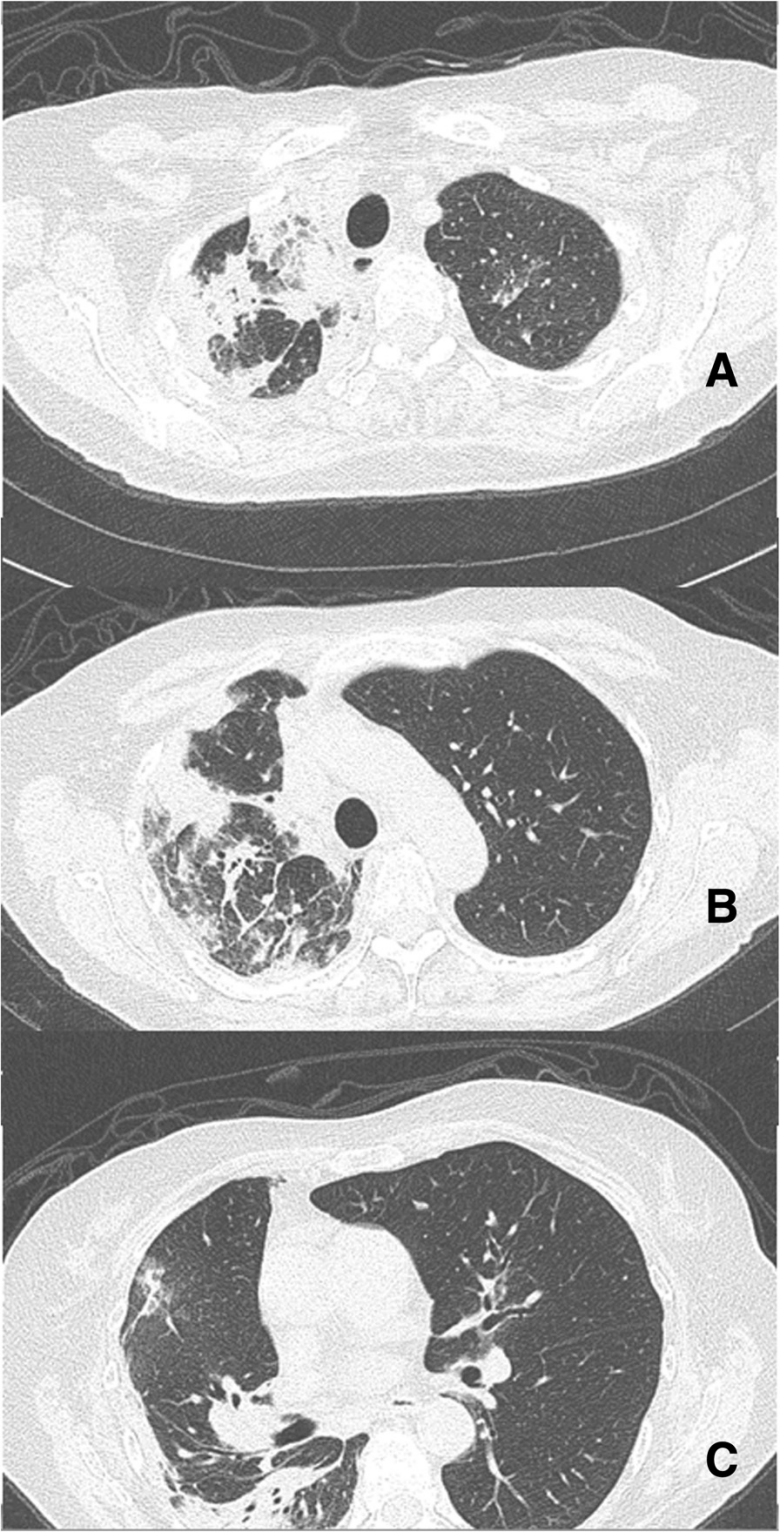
Chest computed tomography showing peripheral pulmonary opacities predominantly in the right lung a apical cut, b mid cut, c lower cut
